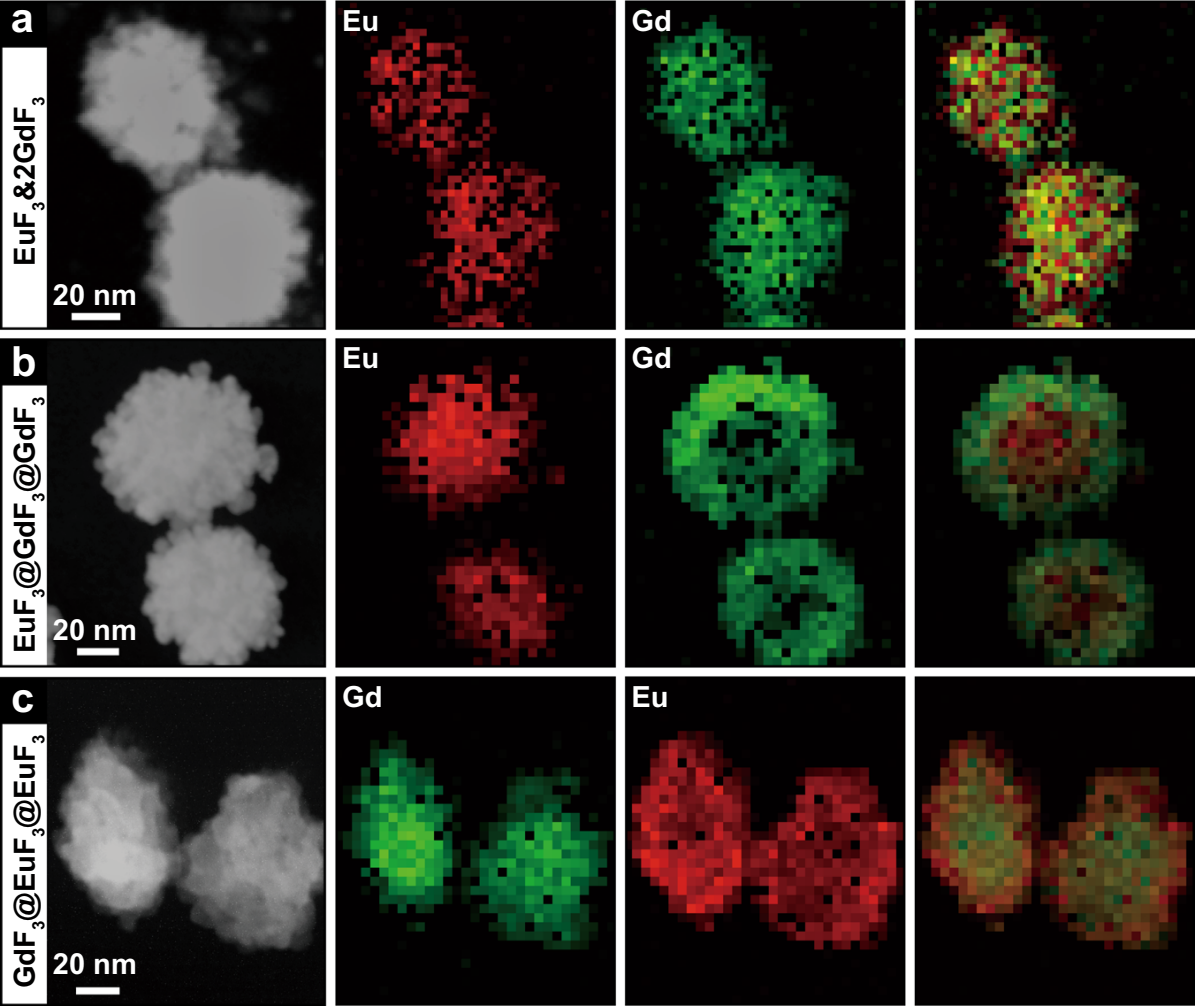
Structural details of different NCs. High-angle annular dark-field scanning TEM images and corresponding energy-dispersive X-ray elemental mapping results of a EuF_3_&2GdF_3_ dimeric, b EuF_3_@GdF_3_@GdF_3_ core-shelled, and c GdF_3_@EuF_3_@EuF_3_ reversely core-shelled NCs.
